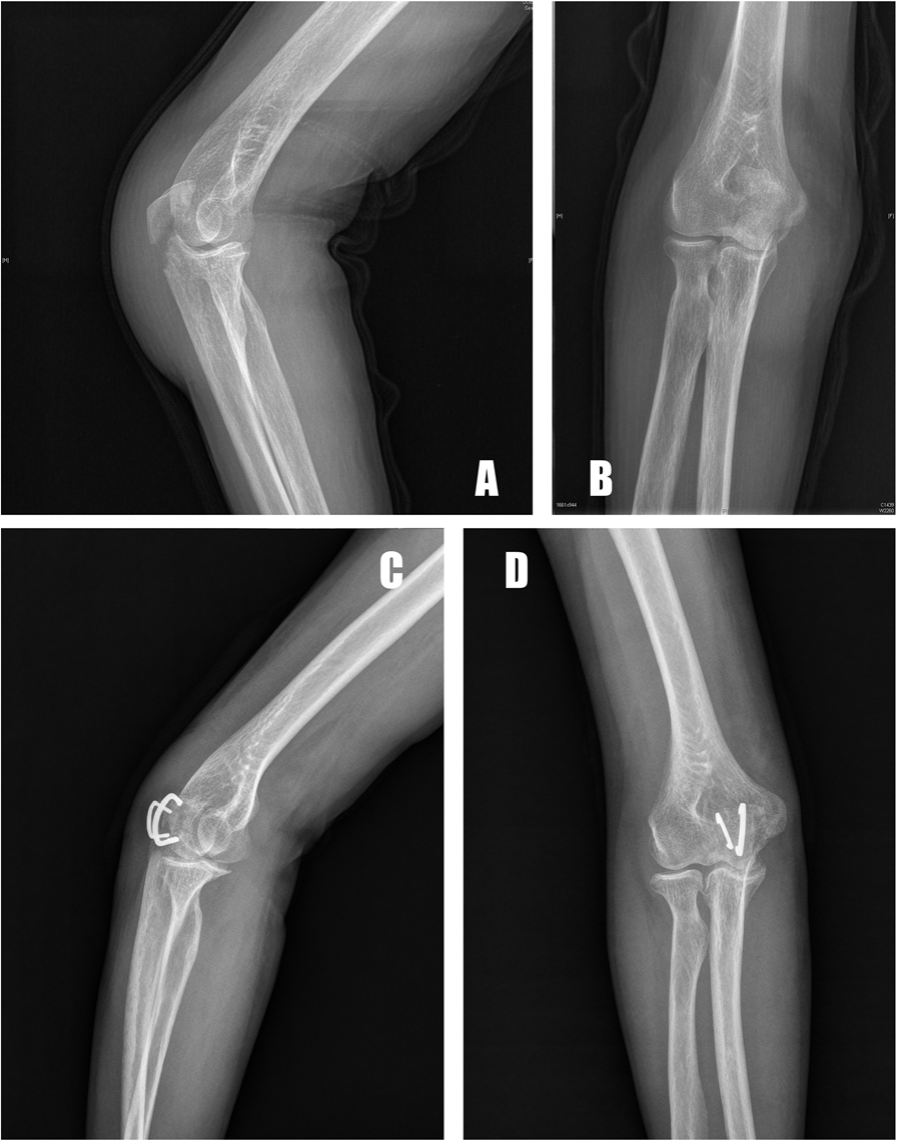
A 54-year-old male patient with comminuted olecranon fracture caused by a vehicular accident (a, b). The comminuted fragments were reduced and fixed anatomically with two ACS. Seven months after surgery, the radiographs showed a healed fracture with metallic implants and no obvious gap or step-off along the joint surface (c, d)
